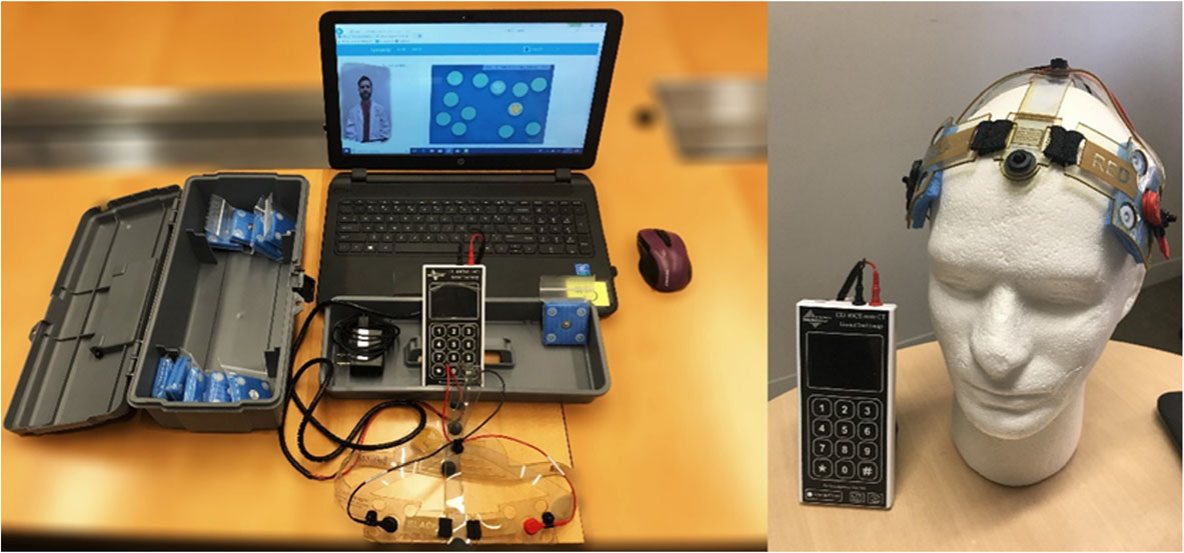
Example of tele-monitored tDCS (tele-tDCS) setup including the tDCS device (for at-home sessions), head strap and the videoconferencing platform. The depicted tDCS device is a Soterix Mini-CT tDCS. The device delivers direct electrical current through saline-soaked sponge electrodes (5 cm × 5 cm) snapped to a custom-made head strap. The head strap has clear labeling for reliable electrode placement (right picture). This configuration provided a uniform bi-hemispheric dorsolateral prefrontal cortex montage centered using a nasion marker. The supervising study technician is shown in the laptop screen (left picture) as a study participant would see it during video-conferencing
